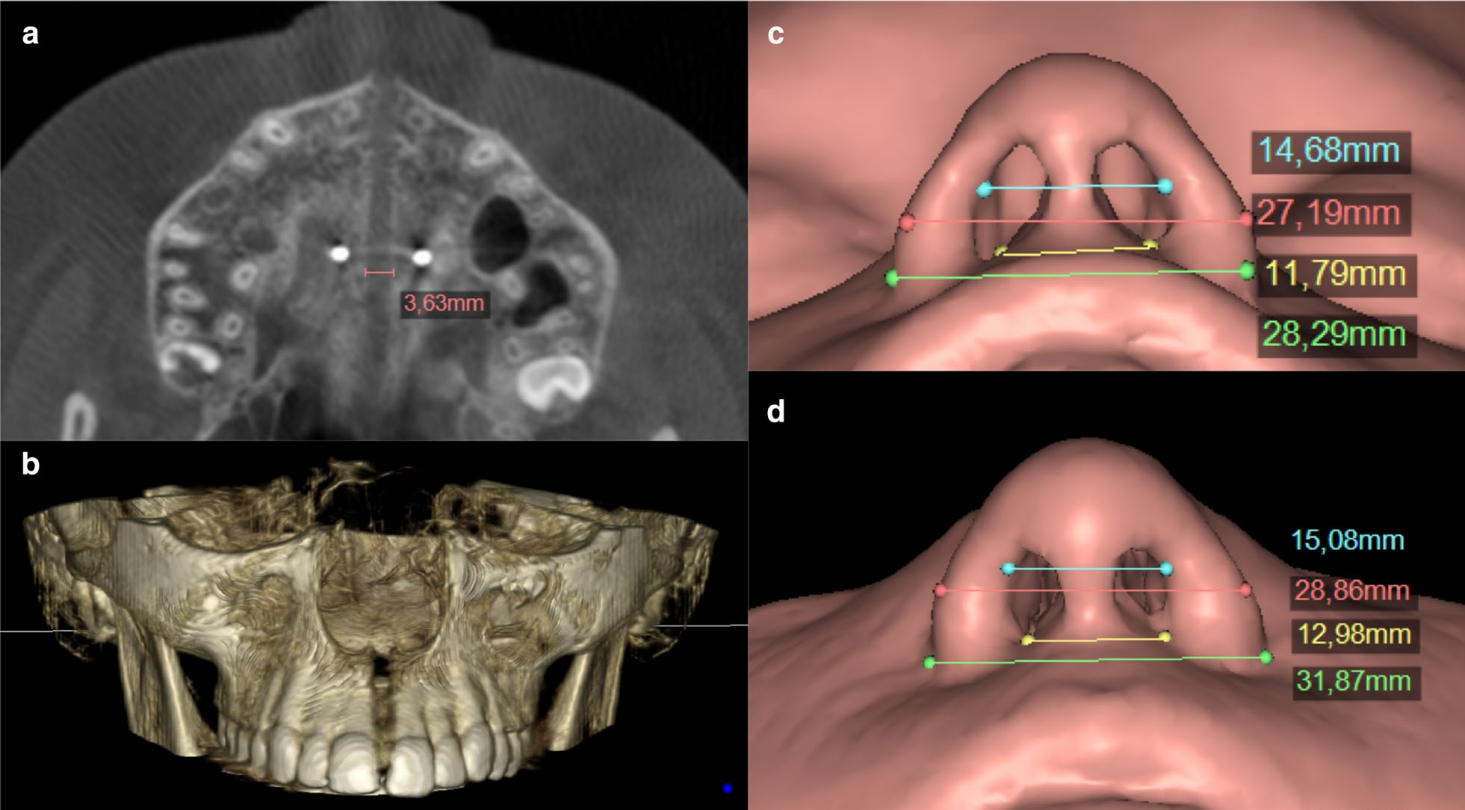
a CBCT occlusal view showing parallel separation of the mid-palatal suture. b 3D composition of the upper maxilla after RME (frontal view). c Landmarks and measurements showing changes of the nose and nostrils before RME. d Nasal morphology changes after RME.
